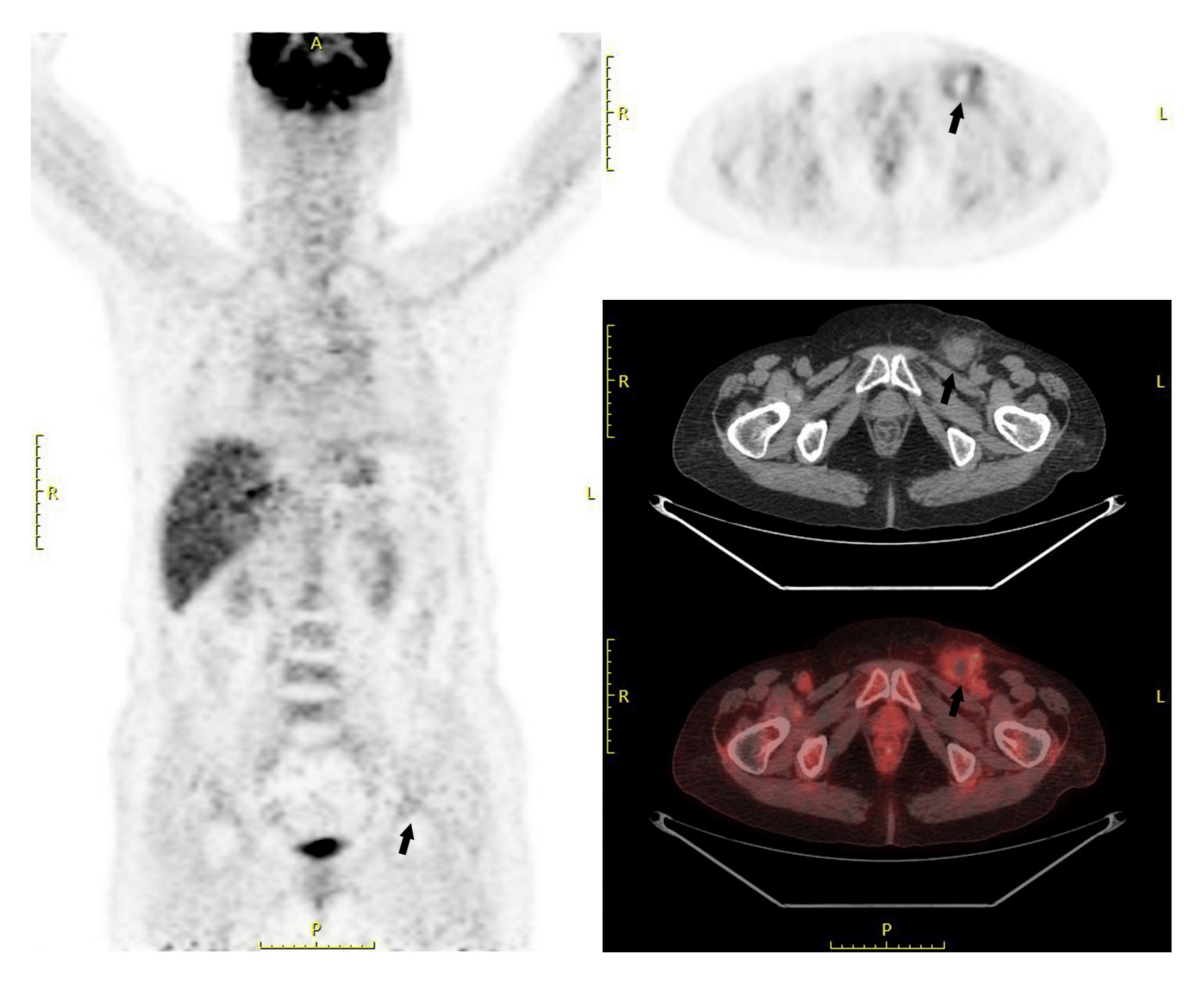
Images of PET-CT scan PET-CT scan showed limited fluorine-18 fluorodeoxyglucose (18F-FDG) uptake in the left inguinal region after the inguinal lymph node biopsy.
